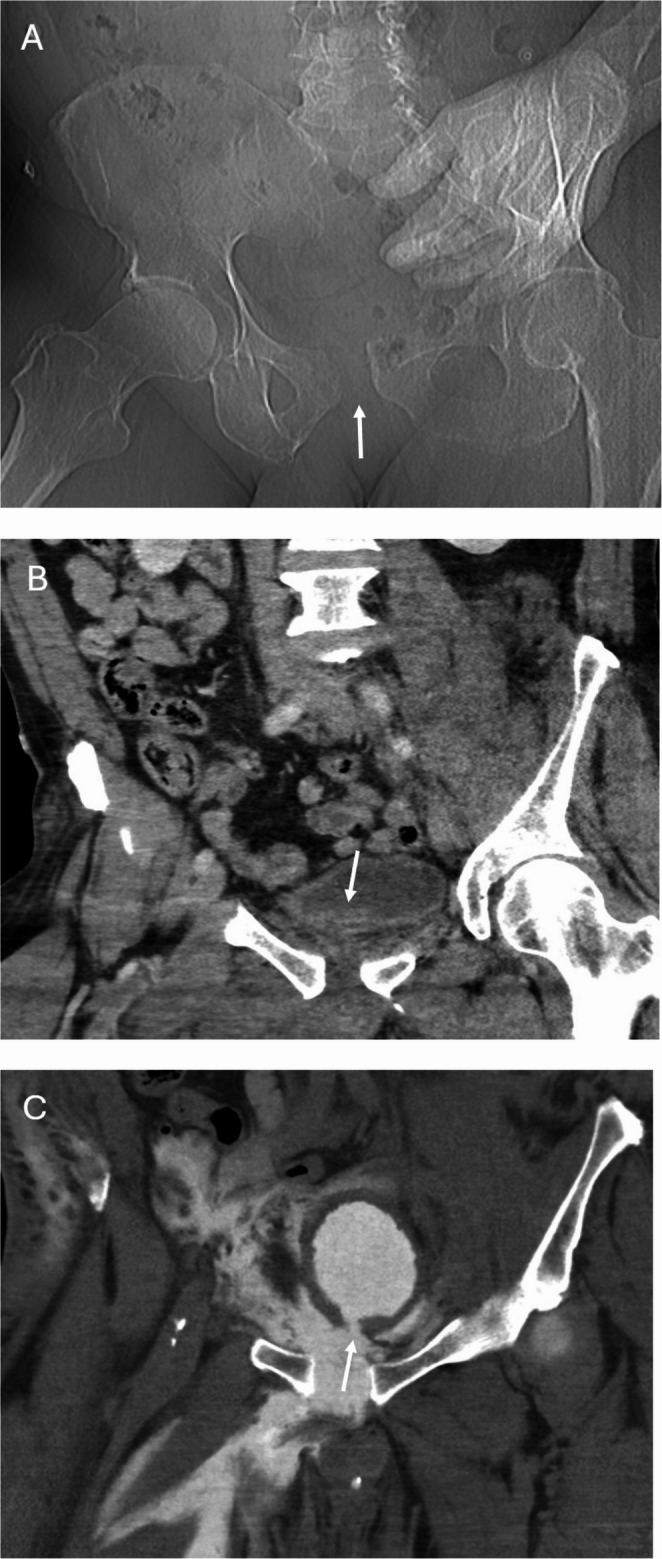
62-year-old male who was struck by a motor vehicle. CT scout image (A) demonstrates abnormal widening of the pubic symphysis. Coronal CT image (B) demonstrates a small hematoma with adjacent bladder wall irregularity (arrow), which was not mentioned in the original outside report. Subsequent coronal CT cystogram (C) images demonstrated a focal defect in the bladder wall (arrow) with leak of a large amount of bladder contrast consistent with an extra-peritoneal bladder injury which was treated non-operatively with bladder decompression
